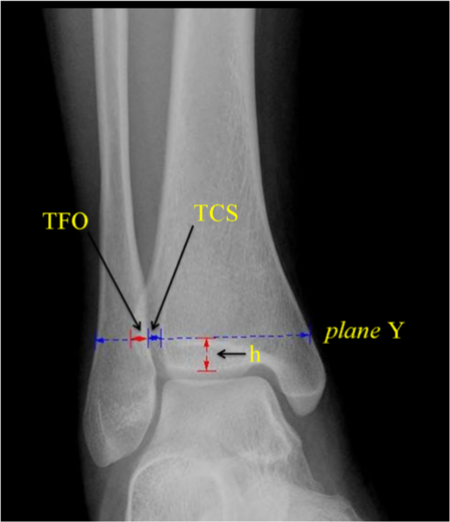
The TCS and TFO in the radiographs were measured at the level of plane Y. The “h” was not invariable, which was the perpendicular distance from plane Y (selected in the 3-D image) to the tibial plafond on the anteroposterior view. TCS = tibiofibular clear space; TFO = tibiofibular overlapThe depth of the incisura fibularis in the 3-D and 2-D images, abbreviated “IFD”, measured the vertical distance from point E to the line between point A and C in the 3-D and 2-D axial images (Fig. 4).The height of the incisura fibularis in the 3-D image and the AP view radiographs, abbreviated “IFH”, measured the vertical distance from point F to plane X (tibial plafond) (Fig. 5).
